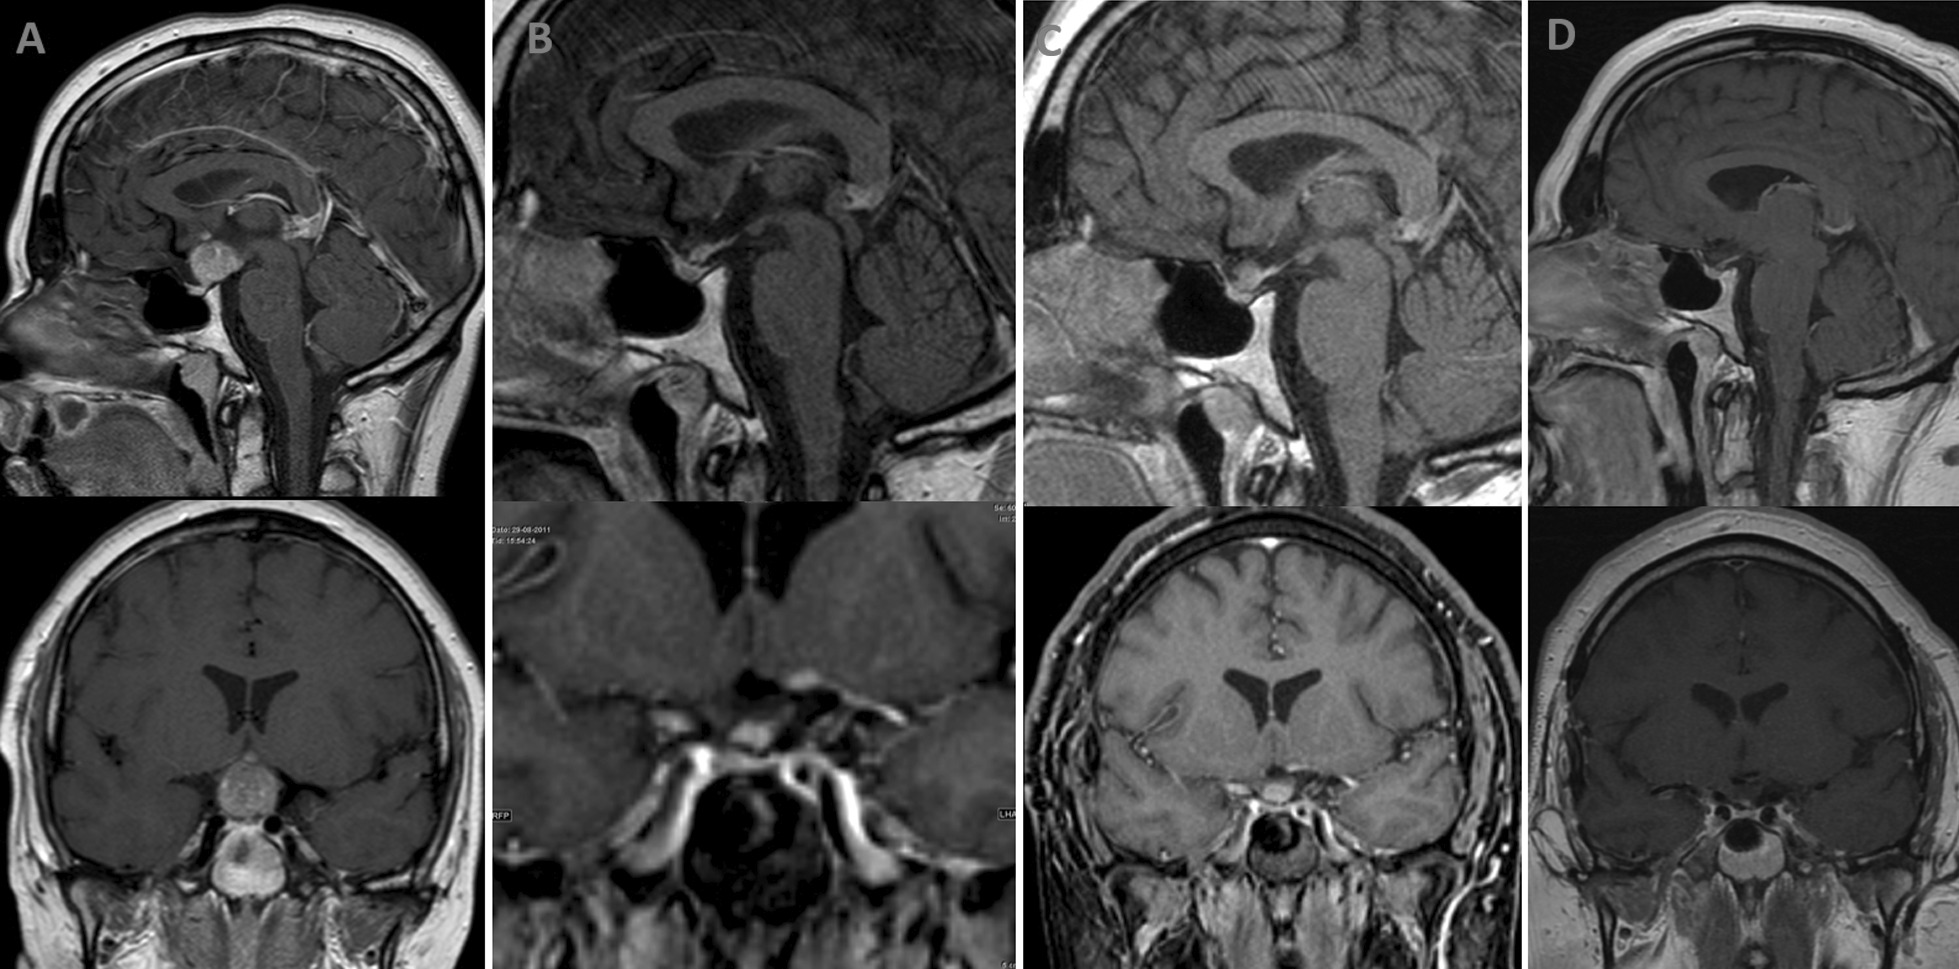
MRI scan showing pituitary enlargement in the T1-weighted sagittal (upper) and coronal section (lower). A Initial MRI before surgery. B Post-surgery with remnant pituitary tissue located just below the optic chiasm. C Relapse under treatment with azathioprine and prednisolone. D Almost complete regression of pituitary mass after rituximab treatment
